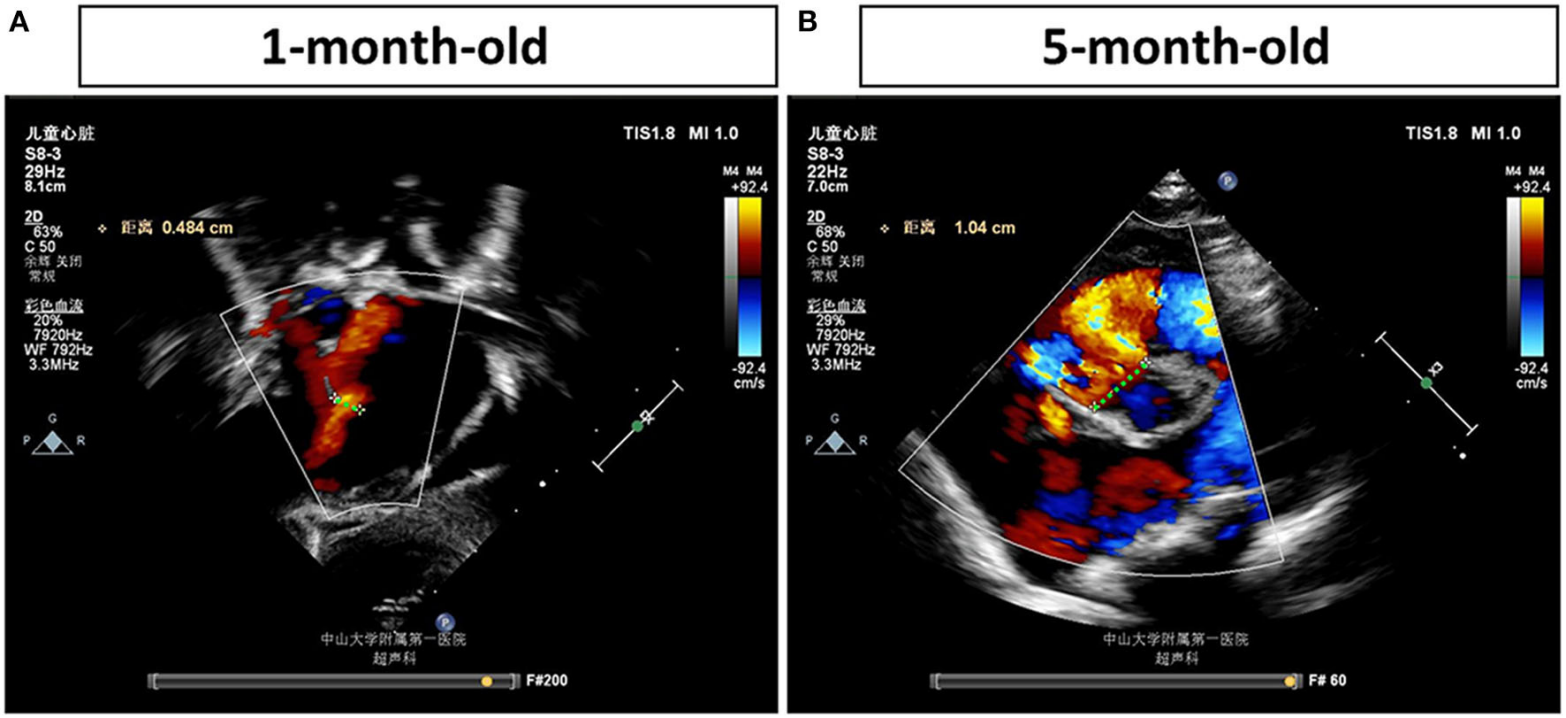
Echocardiogram examinations of the proband at different time points. Representative image of the echocardiogram of the proband's left ventricle obtained in the parasternal long-axis view (A) shows a ventricular septal defect (VSD) (0.484 cm) at 1 month of age. Representative image of the echocardiogram (B) shows a more severe VSD, which is enlarged to 1.04 cm, in the proband's heart at 5 months of age. The largest distances of VSD are marked by green dash lines and measured.
